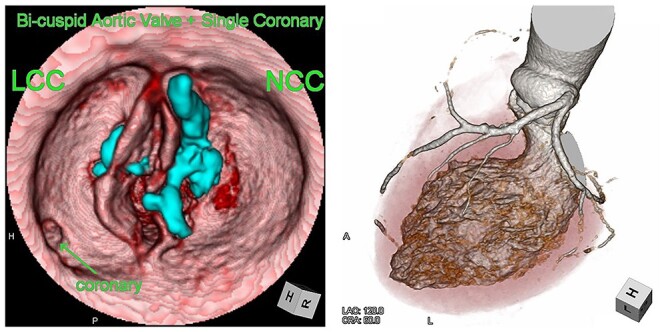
Computed tomography images of the bicuspid aortic valve type 0 and single coronary artery. Heavy calcification on NCC is indicated in blue.
